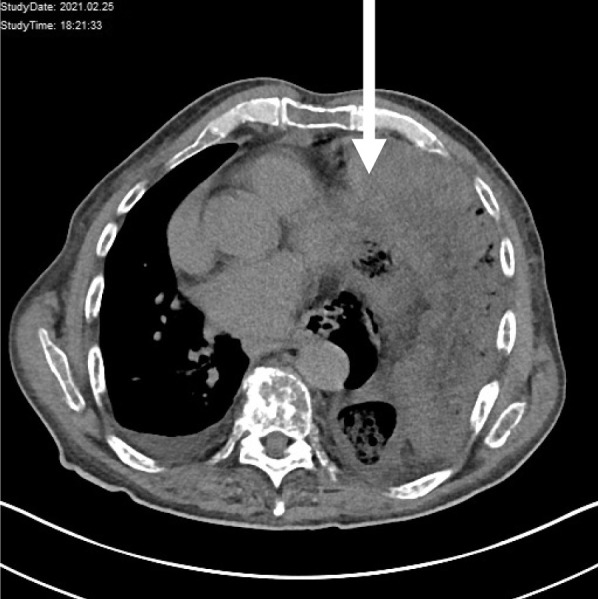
preoperative CT imaging revealed a massive diaphragmatic herniation into the left thorax. As visible on the CT image dextrocardia was present and abdominal organs compressed the left lung resulting in massive dyspnoea of the presented patient (arrow)
